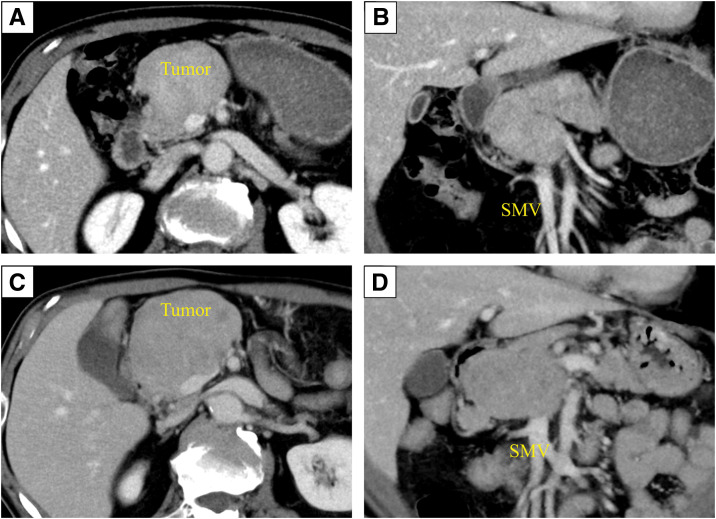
Abdominal CT imaging at the initial diagnosis and after 5 years. (A, B) CT imaging at the initial diagnosis reveals a 61 × 45 mm heterogeneous enhanced tumor in the pancreatic head that does not invade the surrounding tissue but is in contact with the SMV. (C, D) On imaging obtained 5 years after the initial diagnosis, the tumor has grown to 80 × 60 mm without invasion into the surrounding tissue. SMV, superior mesenteric vein
